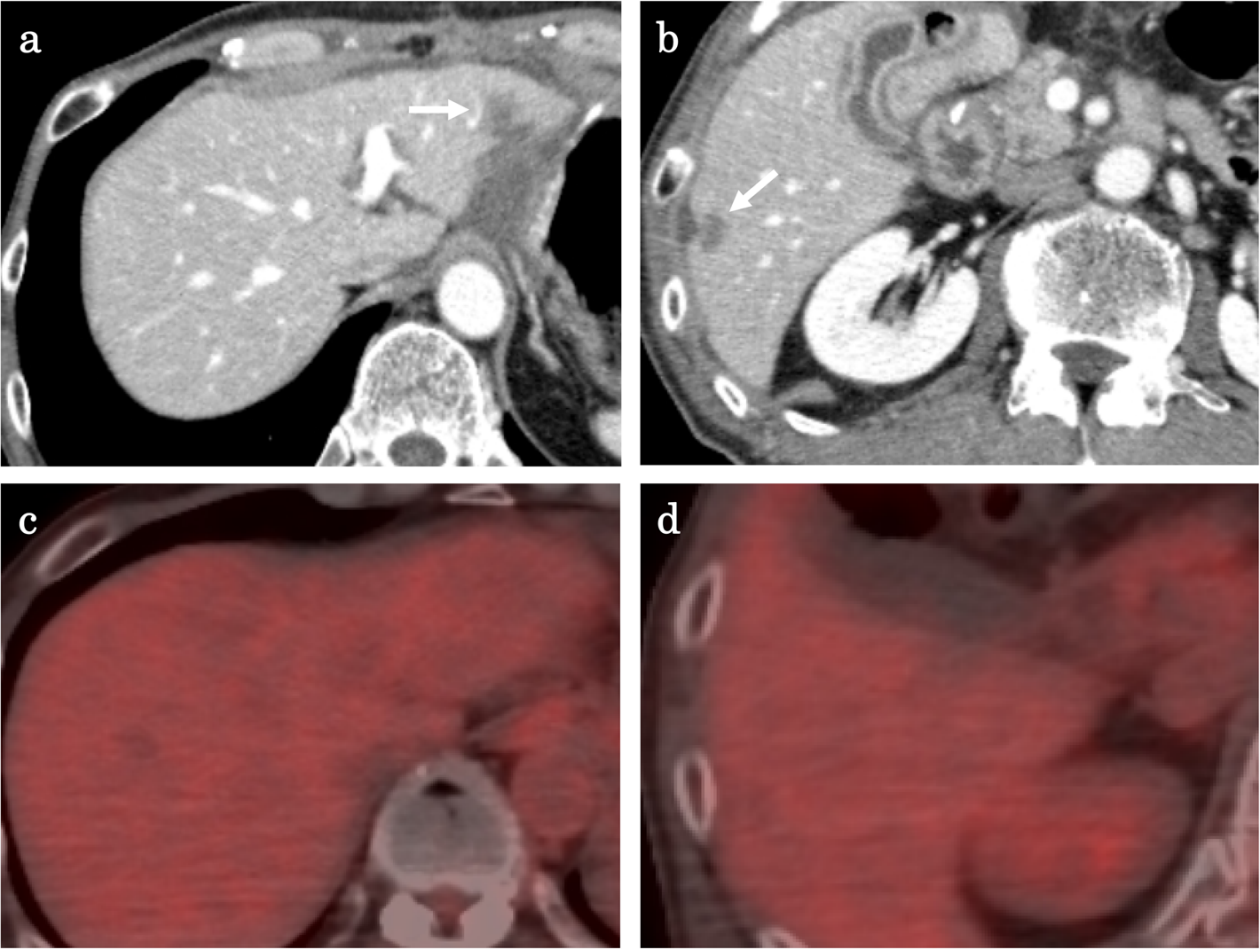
Liver nodules after chemotherapy. The hepatic nodules in segment 2/3 and segment 6 had dramatically reduced in size to 14 mm in diameter (a) and 15 mm in diameter (b), respectively (white arrow). c, d Neither tumor showed 18F-FDG uptake
